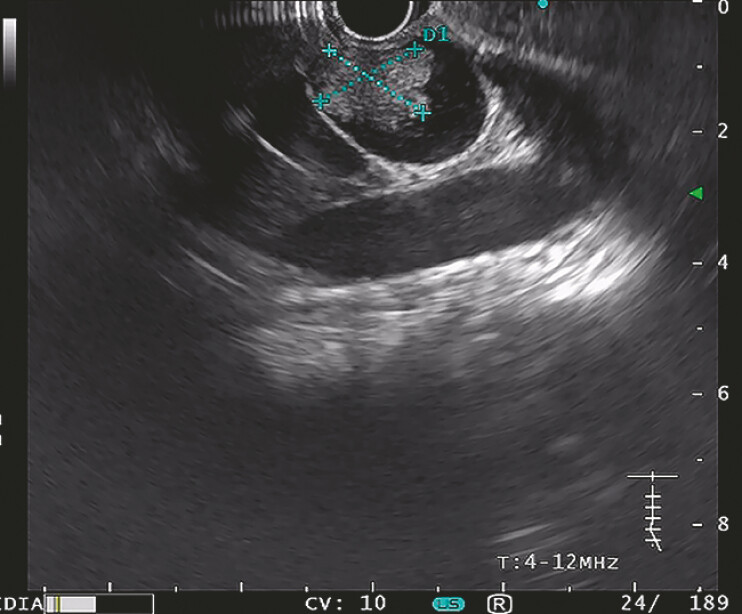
EUS identified a lobulated, hyperechoic lesion (16.5 mm × 17.2 mm) at the remnant common bile duct. Abbreviation: EUS, endoscopic ultrasound.
